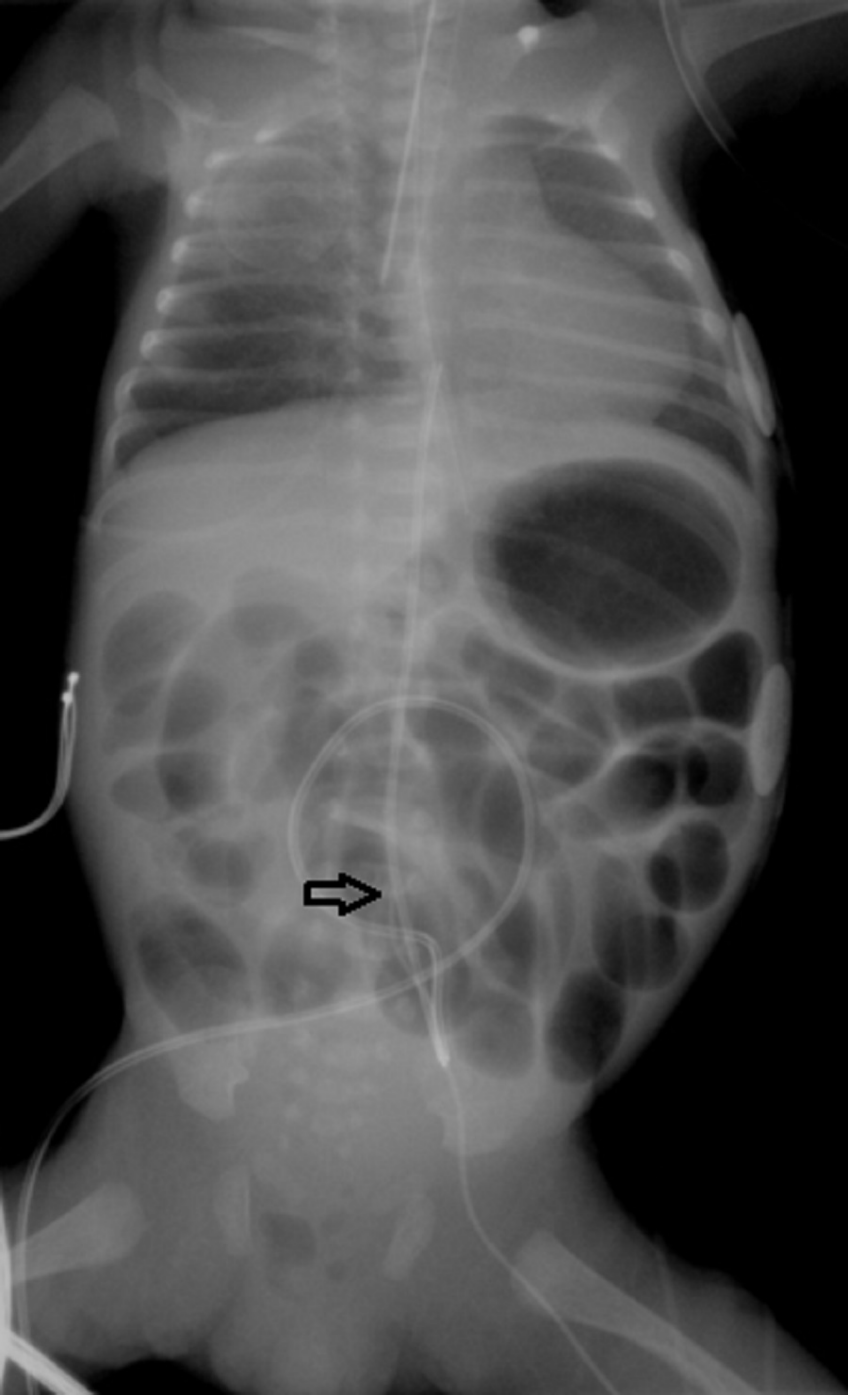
Supine Abdominal Radiograph Demonstrating the left femoral PICC line terminating possibly in a left lumbar vein (Arrow)
